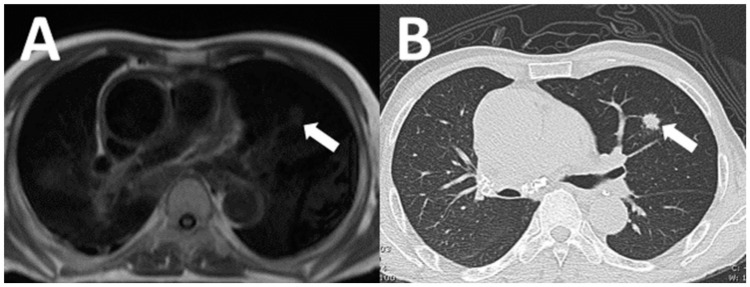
A 72-year-old man underwent CMR to evaluate myocardial viability. Incidentally detected lung nodule on axial black blood image cardiac MRI (A: arrow). Subsequent CT chest demonstrated speculated lung nodule (B: arrow) and was consequently diagnosed as primary lung cancer by CT-guided lung biopsy.
